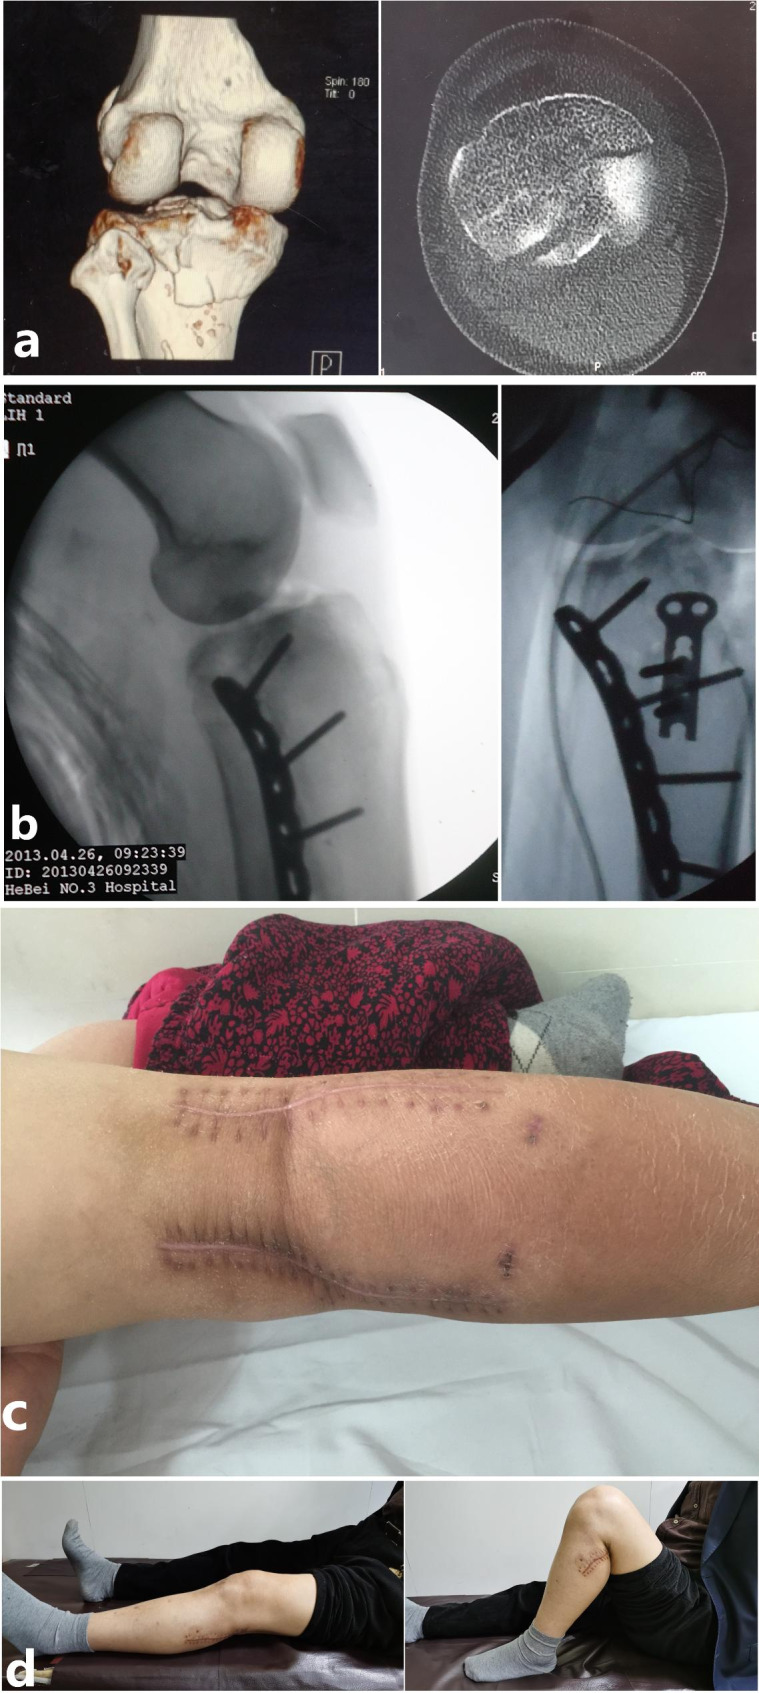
A typical case of a 56-year-old female with Schatzker IV tibial plateau fractures (flexion-valgus-external rotation type). (a) The features of this type were shown in CT images. (b) The reduction and internal fixation of the medial MFA were performed through posteromedial incision first. Then the lateral MCZ was restored and fixed through the posterolateral approach. (c) Bilateral S-shape curvilinear incisions (Carlson Approach) were made for this type of tibial plateau fracture. (d) 1 year postoperatively, the function of the knee was excellent
